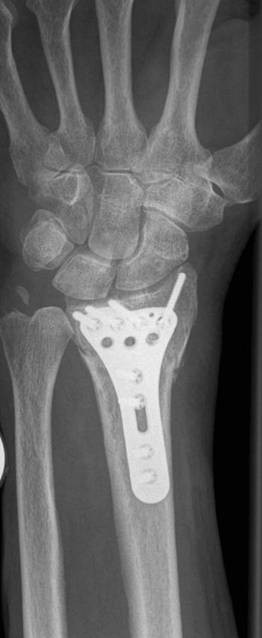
Operative treatment. Postoperative radiograph shows restoration of radial length
